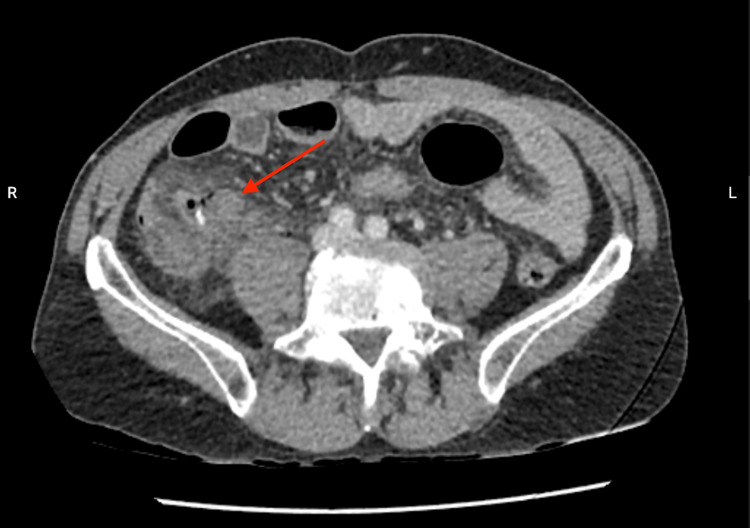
Abdominal CT scan. CT scan showing fat infiltration around the appendix (red arrow).
